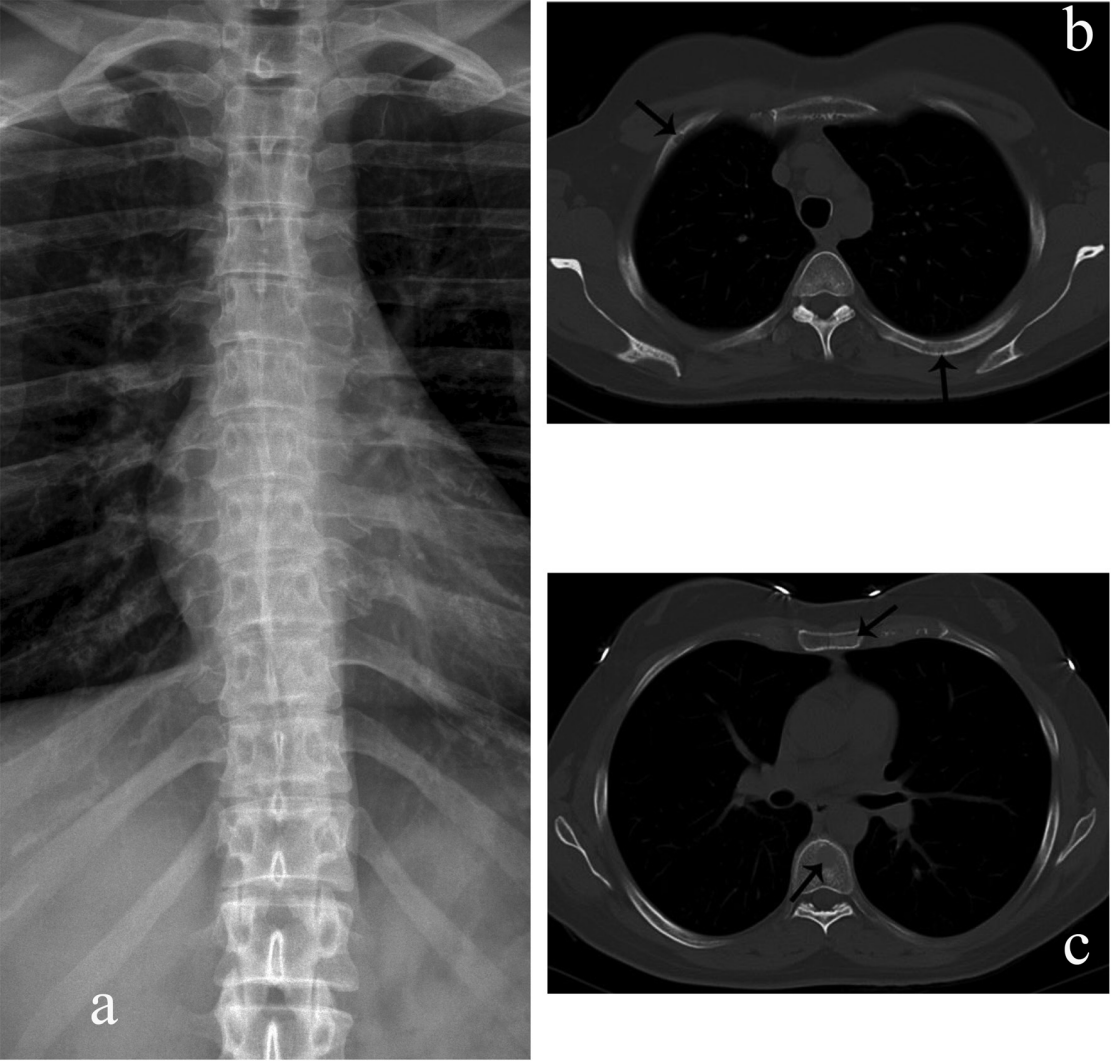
Rib, sternal, and vertebral body myelomatous lesions seen by WBCT (arrows) (b, c) but not conventional skeletal radiography (a).
